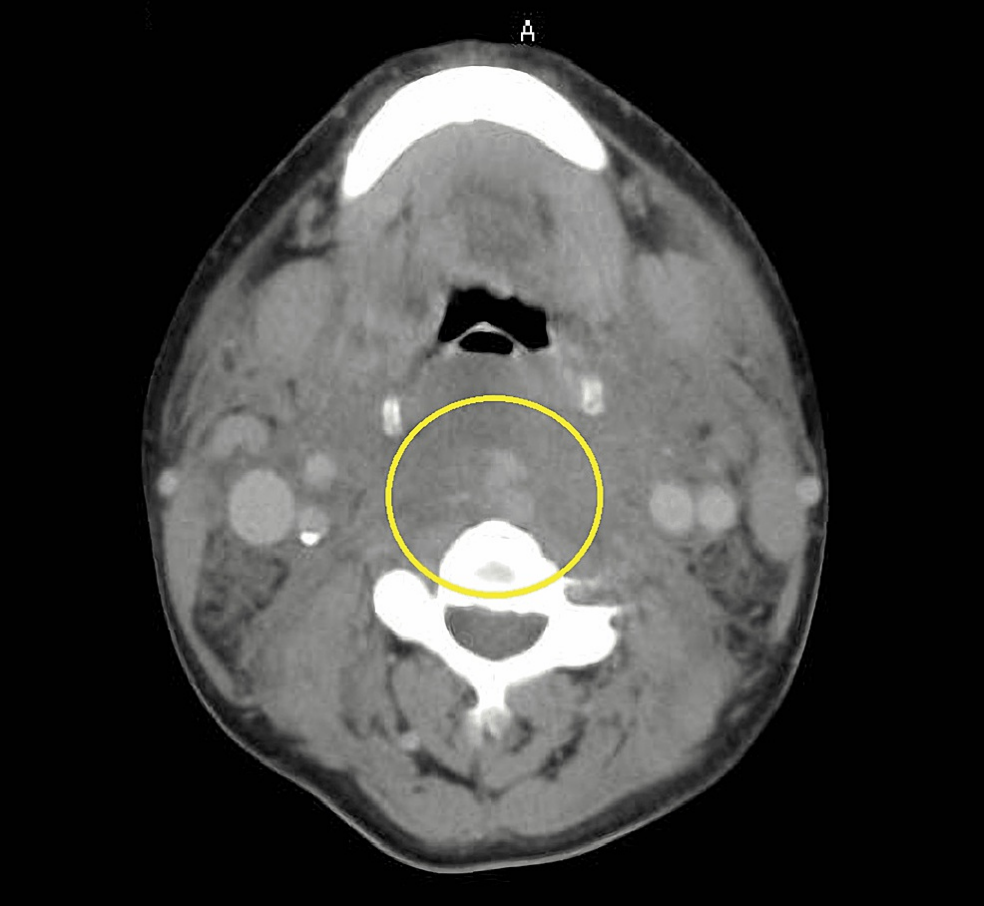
Cervical contrast CT at initial examination (axial) Swelling of the retropharynx is noted.
